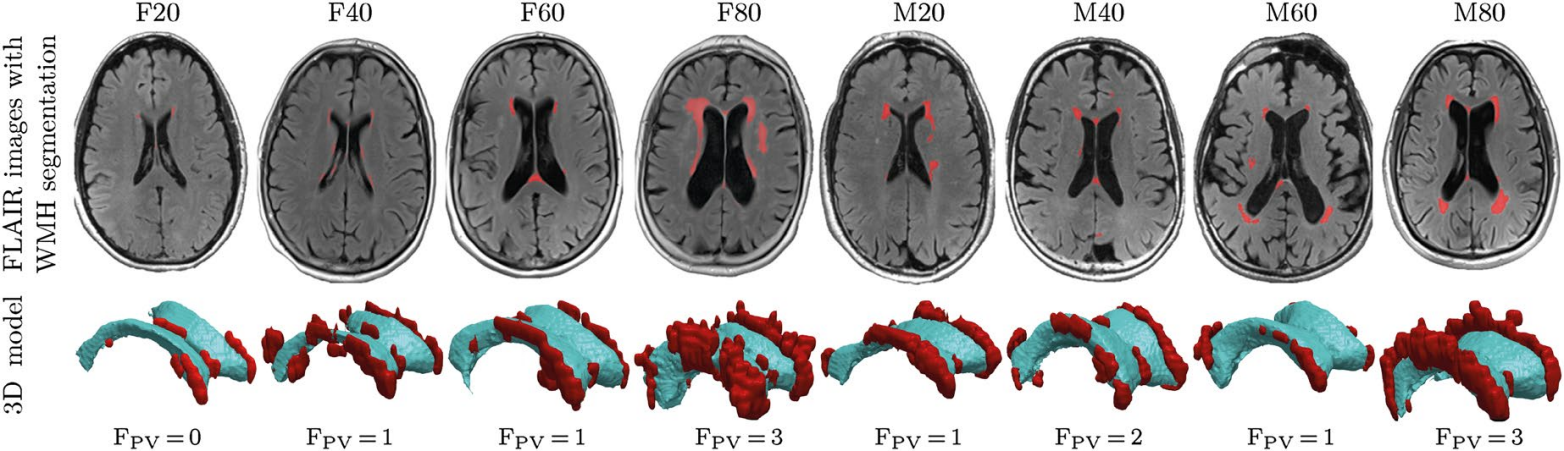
Representative axial slices and volumetric reconstructions of lateral ventricles and white matter hyperintensities from eight subjects. We sorted 464 subjects from the NYU ADRC’s imaging database based on their total intracranial CSF volume and picked a male and female subject from the 20th, 40th, 60th, and 80th percentile. The top row shows the FLAIR MRI sequence of all eight patients with a white matter hyperintensity mask (red); the bottom row shows volumetric reconstructions of their ventricles (blue) and white matter hyperintensities (red). We also provide the Fazekas score of the periventricular WMHs.
